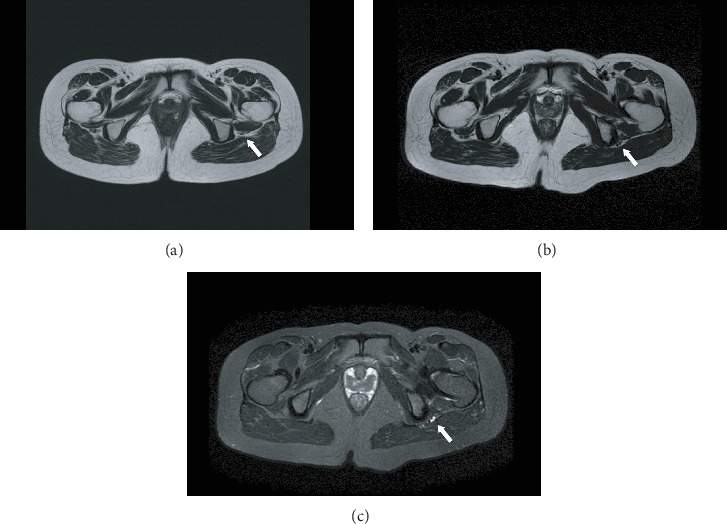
Preoperative and postoperative magnetic resonance imaging. (a) T2-weighted images of preoperative magnetic resonance imaging. The arrow indicates the sciatic nerve and soft tissue without degeneration. (b) T2-weighted images of 12 months postoperative magnetic resonance imaging after left-side curved periacetabular osteotomy. The arrow indicates denatured soft tissue around the sciatic nerve. (c) Short T1 inversion recovery images of 12 months postoperative magnetic resonance imaging after left-side curved periacetabular osteotomy. The arrow indicates intensity change in the sciatic nerve, suggesting nerve degeneration.
